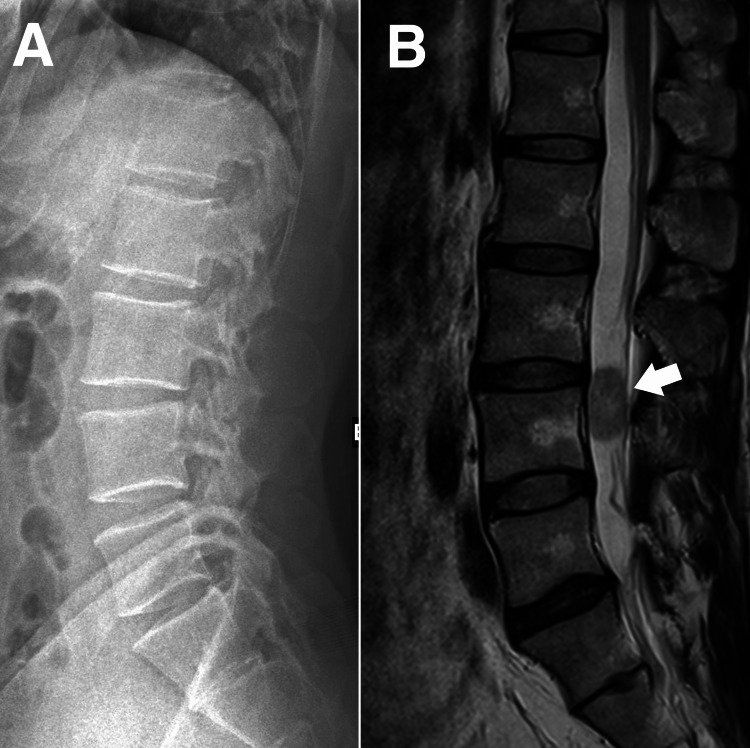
Radiological examinations of the lumbar spine. (A) Initial lumbar radiograph identified mild degenerative joint disease at the lumbar spine. (B) Six months after the initial onset, the chiropractor requested a magnetic resonance imaging of the lumbar spine, which revealed a suspicious oval T2 mild hypointense intrathecal mass at the L4 level, measuring 1.89 cm x 1.16 cm x 2.39 cm (white arrow). The nerve roots are compressed and displaced peripherally.
